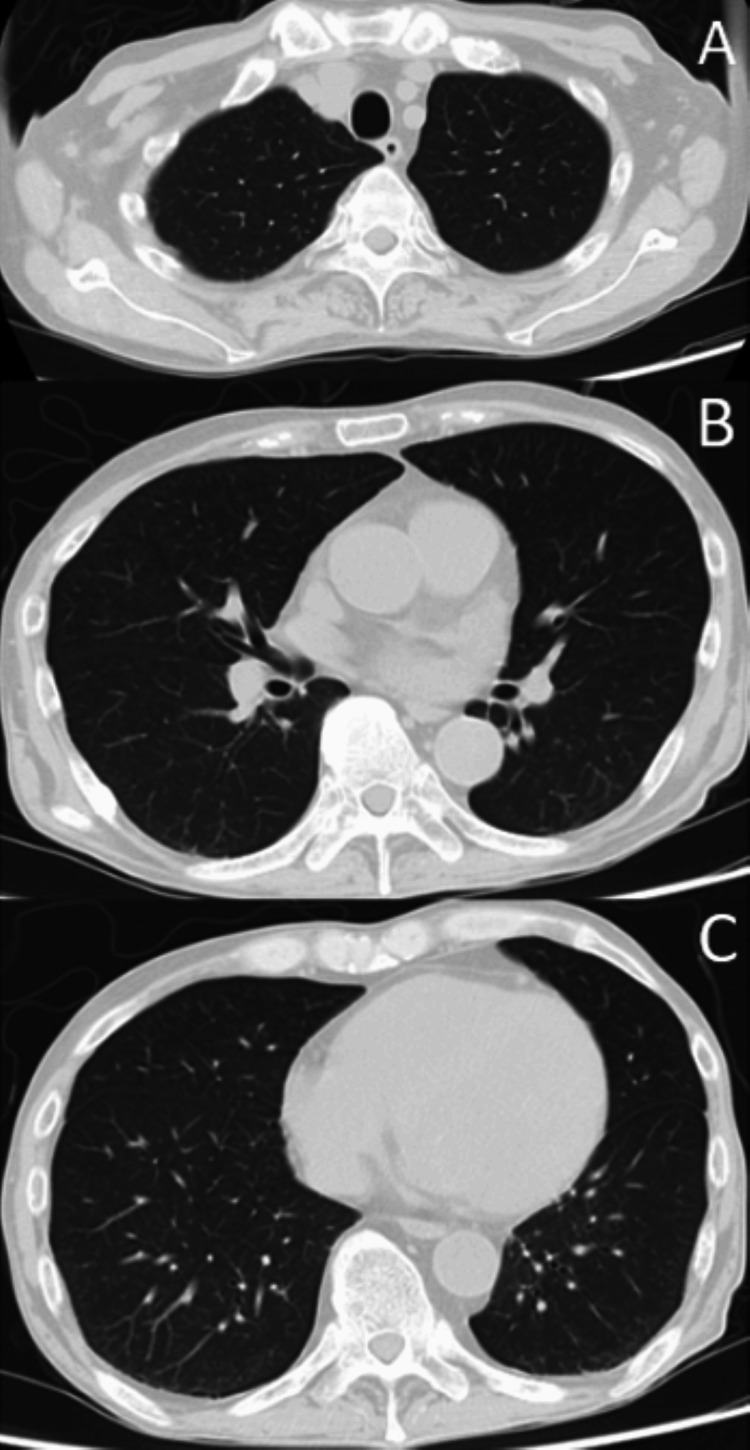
Axial chest CT image at (A) the upper lung fields, (B) the mid-thorax, and (C) the lower lung fields The chest CT scan at admission shows no signs of consolidation or pleural effusion.
